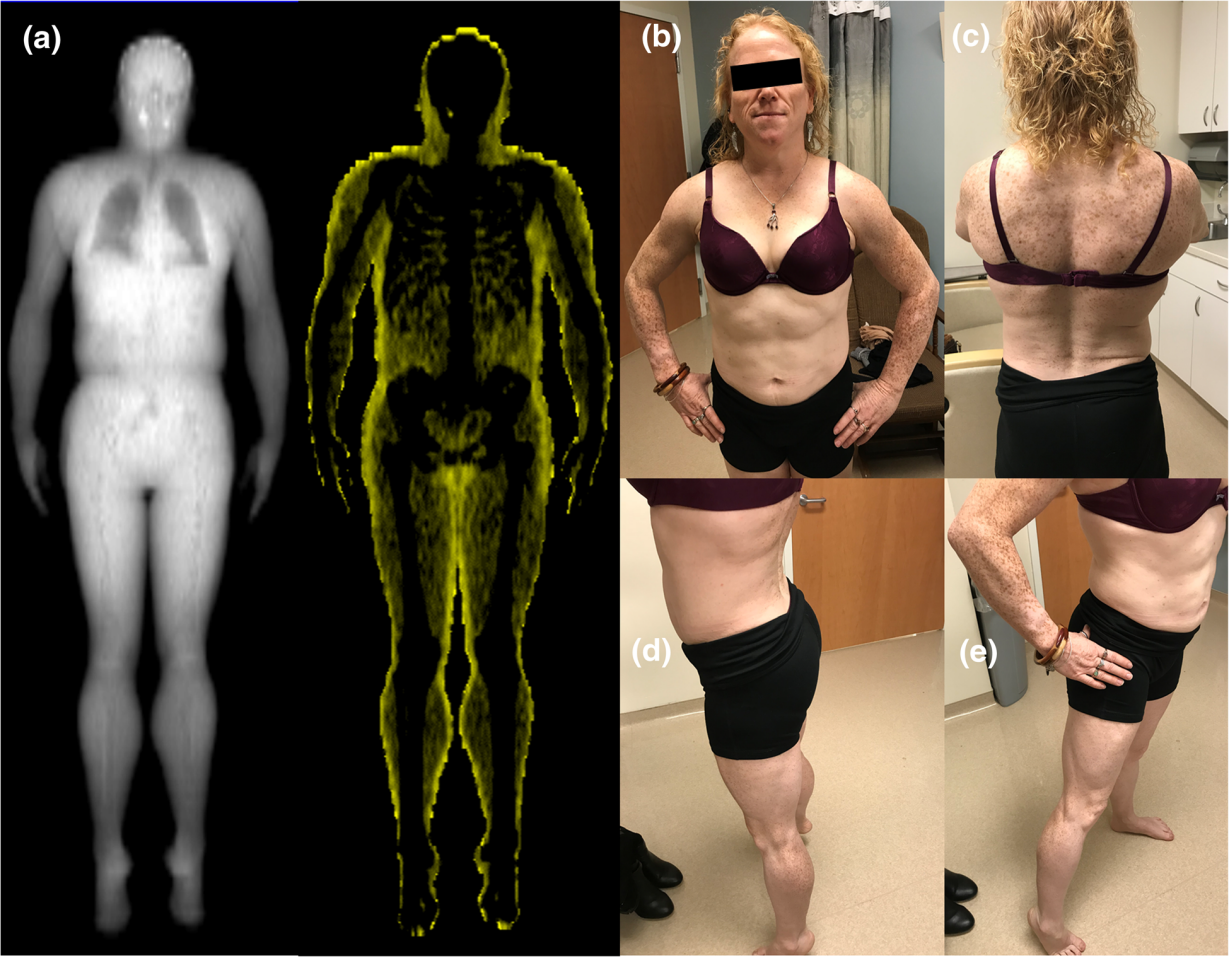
Clinical Examination of Fat Distribution. Panel (a) show the “Fat Shadow” obtained from Dual X-ray Energy absorbtiometry (DXA) scan as described previously in Reference [10]. The fat shadow shows minimally retained fat around the neck and axilla, but loss of fat in a generalized fashion though not totally absent from the body. Close-up pictures taken in clinic setting demonstrate fat loss in the face and trunk (b), back (c), buttock and back of the legs (d), and forearms and legs (e). The absence of fat from the face together with the entire abdomen, trunk and extremities favor the diagnosis of generalized lipodystrophy as opposed to partial lipodystrophy. Neck fat is preserved, but not excessive. Mons pubis fat was not increased
